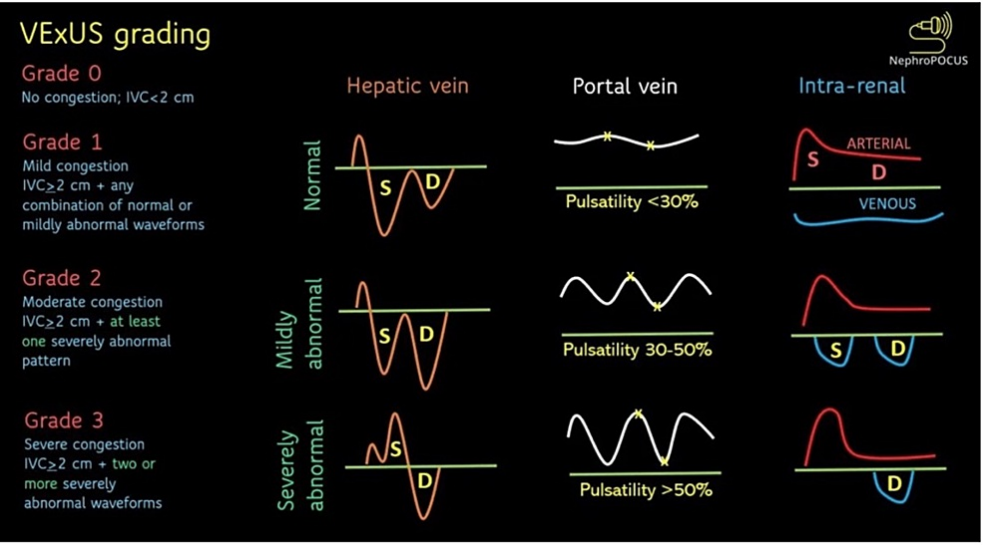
Vascular Doppler ultrasound of abdominal organs Source: Koratala and Reisinger, 2022 [21]. Reprinted with permission. VExUS: venous excess ultrasound; IVC: inferior vena cava
